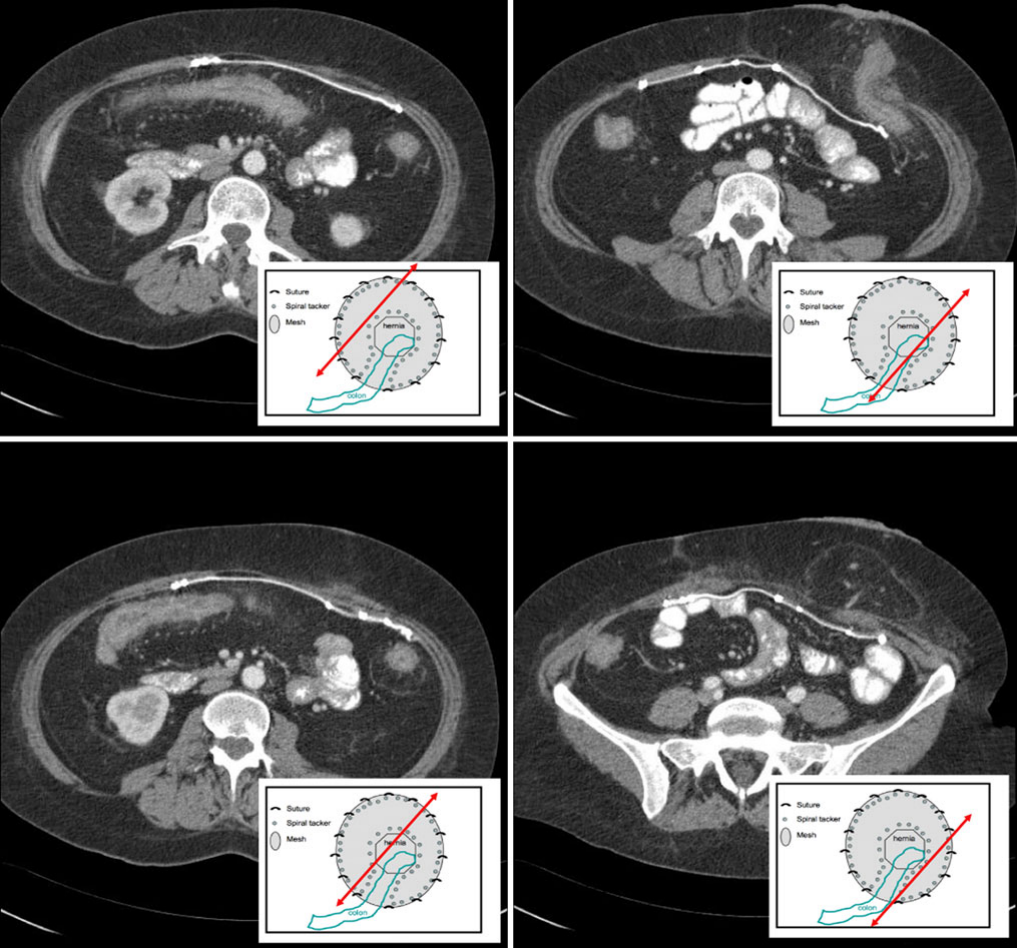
Postoperative multislice CT scan after laparoscopic Sugarbaker repair
